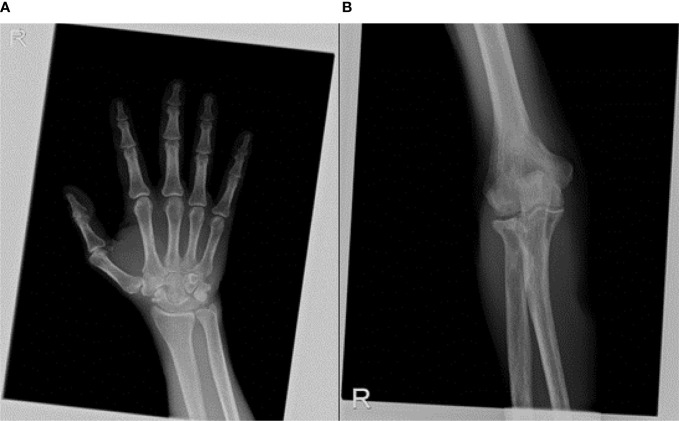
X-ray of articular sites, such as wrist (A) and elbow (B) showed a cartilaginous deposit of calcium pyrophosphate, as for chondrocalcinosis.
